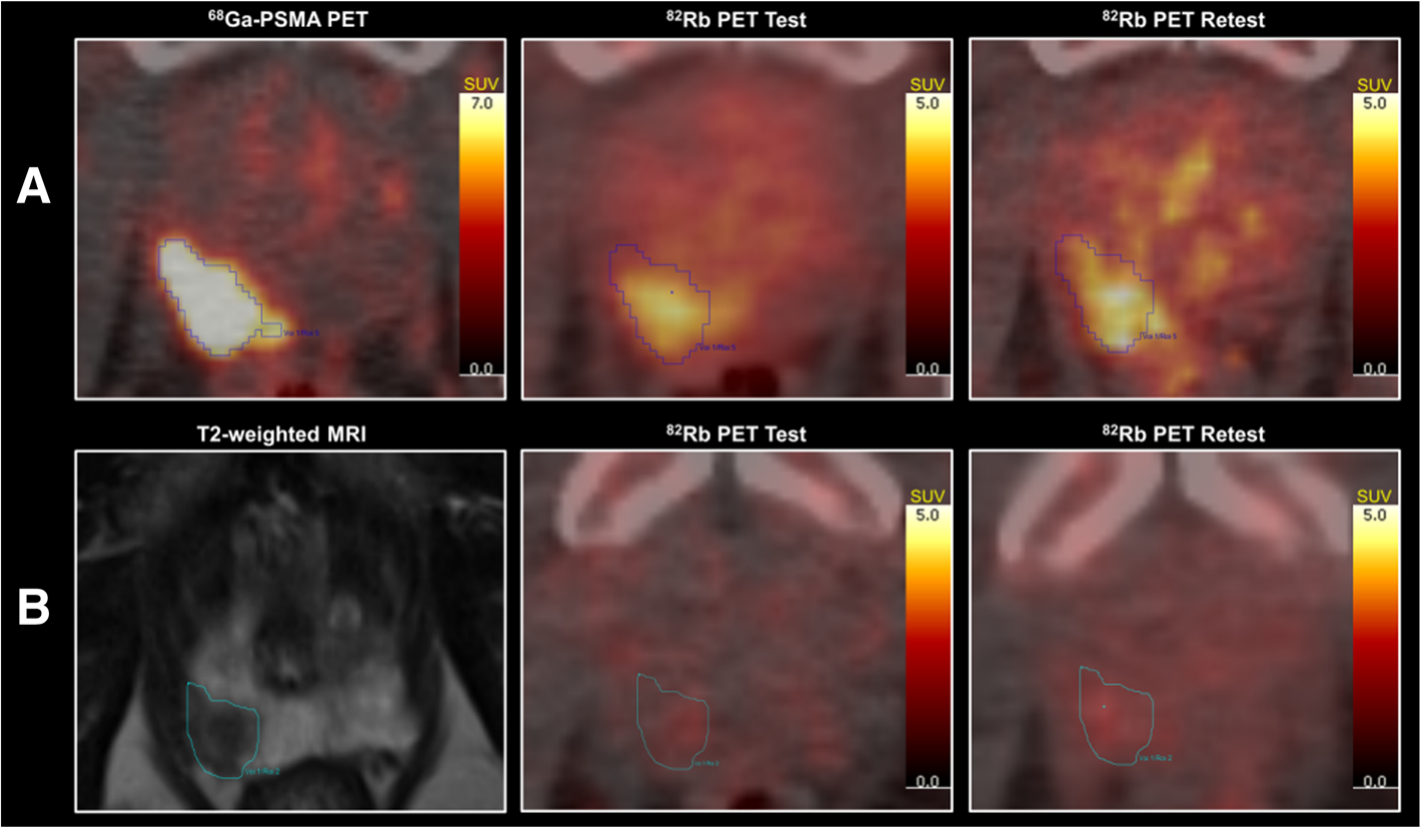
Images of two patients in the study. a Patient 2, a high-risk patient with a Gleason 5+4 high-flow tumor and metastatic disease. To the left, ^68^Ga-prostate-specific membrane antigen (PSMA) positron emission tomography (PET)/computed tomography (CT) with automatically drawn volumes of interest (VOIs) from standard uptake value (SUV) 6 fixed threshold in blue. The first ^82^Rb PET/CT scan in the middle and the second scan to the right. b Patient 9, a low-risk patient in active surveillance with a low-flow tumor. To the left, t2-weighted magnetic resonance imaging with hand-drawn VOIs in light blue. The first ^82^Rb PET/CT scan in the middle and the second scan to the right
